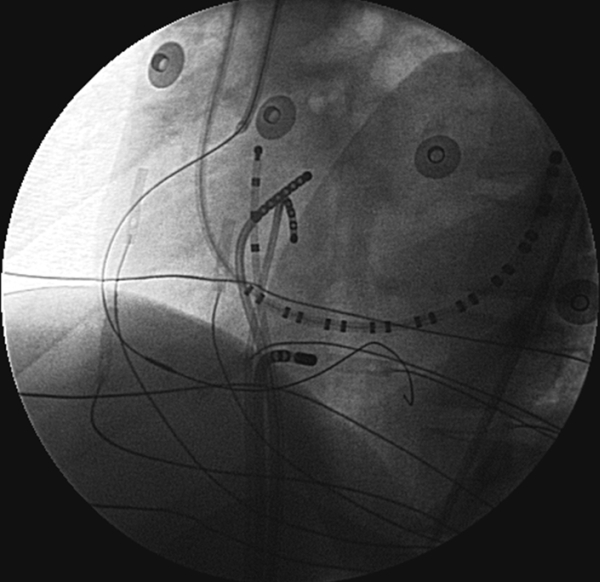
Left anterior oblique fluoroscopic image showing an ablation catheter placed near the ostium of the middle cardiac vein.  A guiding sheath has engaged the right coronary artery and an intravascular ultrasound probe placed into the arterial system.  The probe can be moved so as to image the relevant heart rate when applying radiofrequency energy within a proximate vein.
